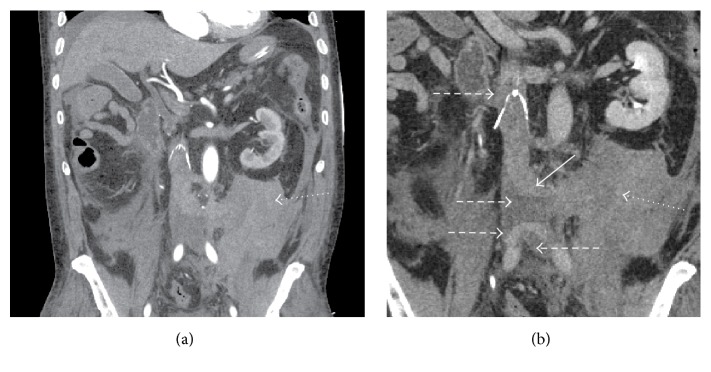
(a) Subsequent contrast CT coronal reformatted image during arterial phase demonstrated no active aortic or arterial contrast extravasation associated with a high density large retroperitoneal hematoma (dotted arrow). (b) Contrast CT coronal reformatted image during delayed (120 sec) venous phase demonstrated hyperattenuated contrast material below the IVC filter contiguous with dilated left lumbar vein (arrow) extending to left retroperitoneal hematoma (dotted arrow). Nonocclusive hypoattenuated clots (dashed arrows) are present below the IVC filter along with an irregular contour of the distal left IVC. Small nonocclusive thrombi above the IVC filter tip and in the iliac veins are also appreciated (dashed arrows).
